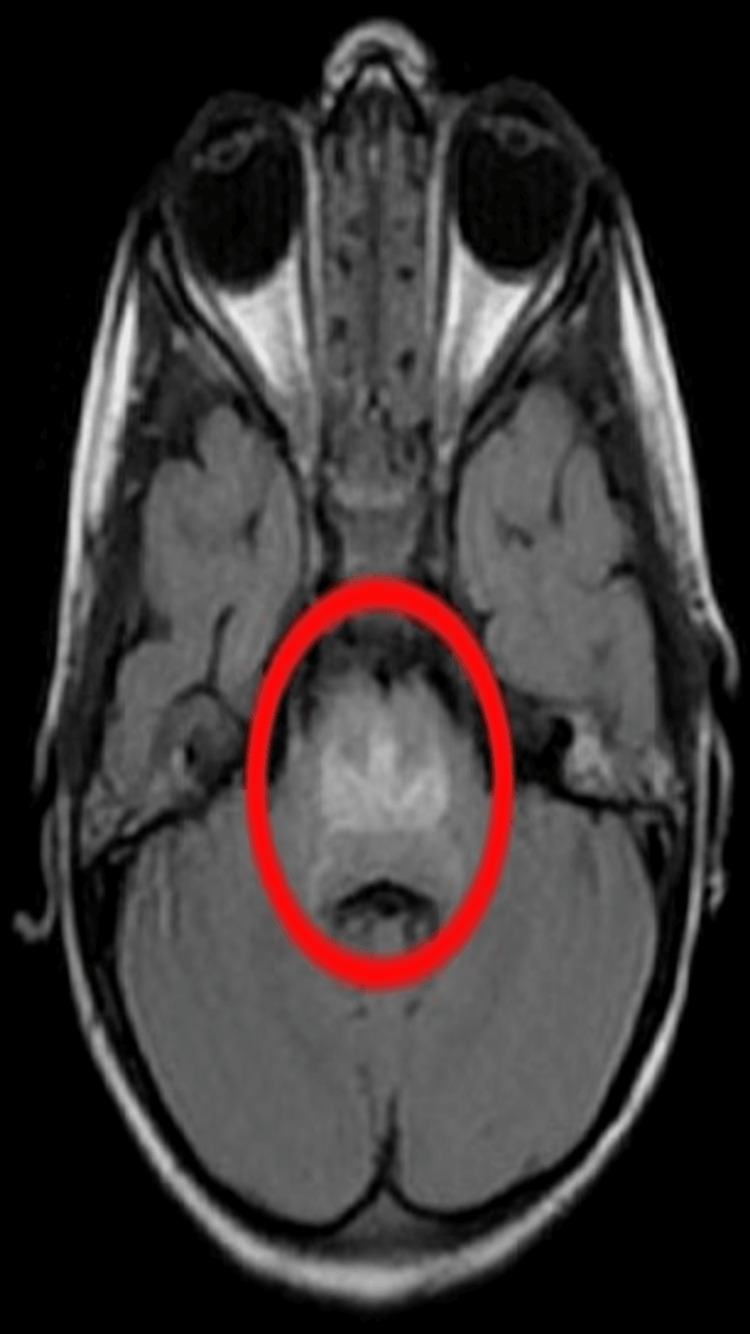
MRI of the brain (the focal lesions in the pons are indicated in the red circle)
